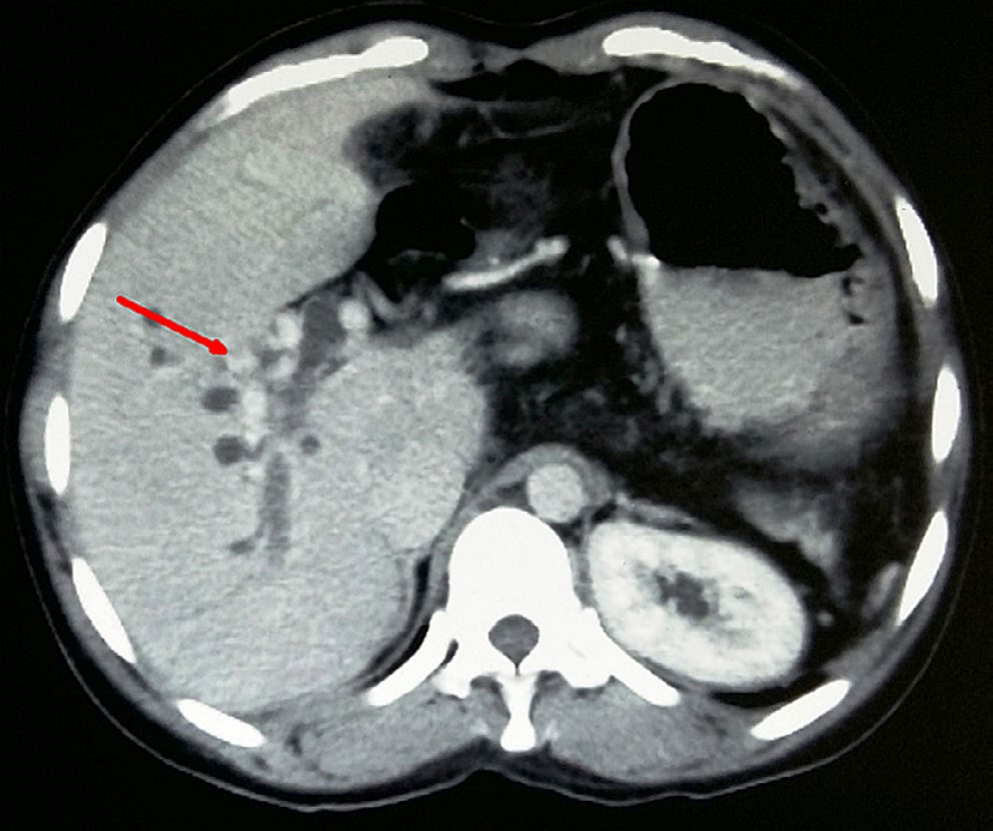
Contrast‐enhanced CT abdomen showing cavernomatous transformation (arrow) of main portal vein with intrahepatic bile duct dilatation and absence of spleen on the left side
